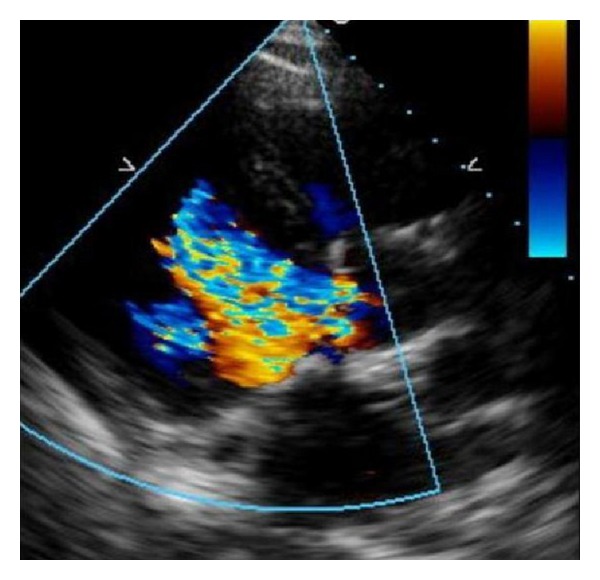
Transthoracic echocardiogram. Parasternal long-axis view reveals aortic regurgitation with both central and peripheral jets suggestive of valve perforation.
